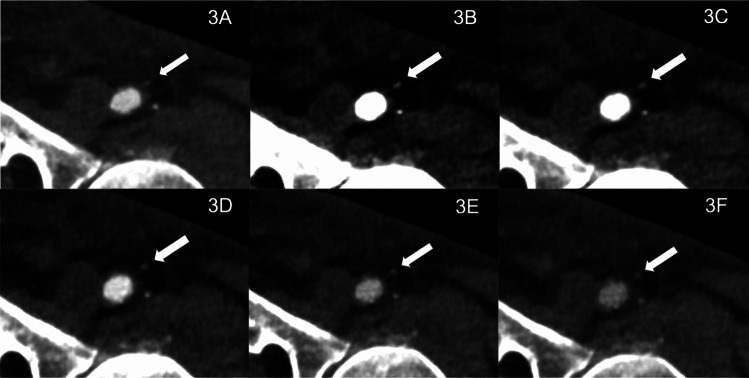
Photon-counting CT angiography in a 65-year-old male with aortic valve stenosis Pre- Transcatheter Aortic Valve Implantation (TAVI): Utilizing 75 ml of 320 mg/mL iodixanol (Visipaque, GE Healthcare) at 3.5 ml/second. Displayed are T3D reconstructions (A) and monoenergetic 40–100 keV reconstructions in 15 keV intervals (B–F) of the left common femoral artery (CFA). T3D (polychromatic) reconstructions showed no vascular pathology. Low-keV reconstructions provided an increase in CNR, peaking at 40 keV VMI (virtual monoenergetic imaging). Optimal image quality for interpretation was noted at 55–70 keV. A smaller artery superficial to the CFA (arrow), potentially at risk during TAVI sheath insertion, was masked at T3D but could clearly be delineated in 40 and 55 keV VMI reconstructions, underscoring the need for careful navigation to avoid inguinal hemorrhage, a common TAVI complication
